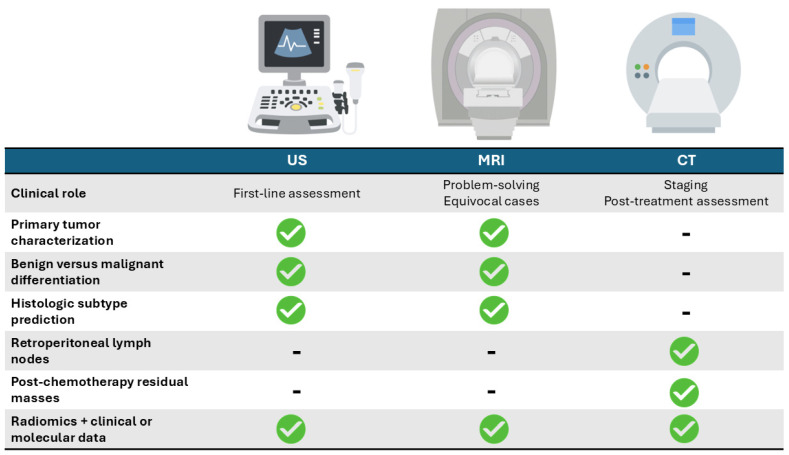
Conceptual overview of radiomics and artificial intelligence applications in testicular cancer imaging across ultrasound (US), magnetic resonance imaging (MRI), and computed tomography (CT). Checkmarks indicate clinical contexts addressed by radiomics-based approaches in the included studies, while dashes indicate applications not evaluated. The first row summarizes the typical clinical role of each imaging modality within the diagnostic pathway.
